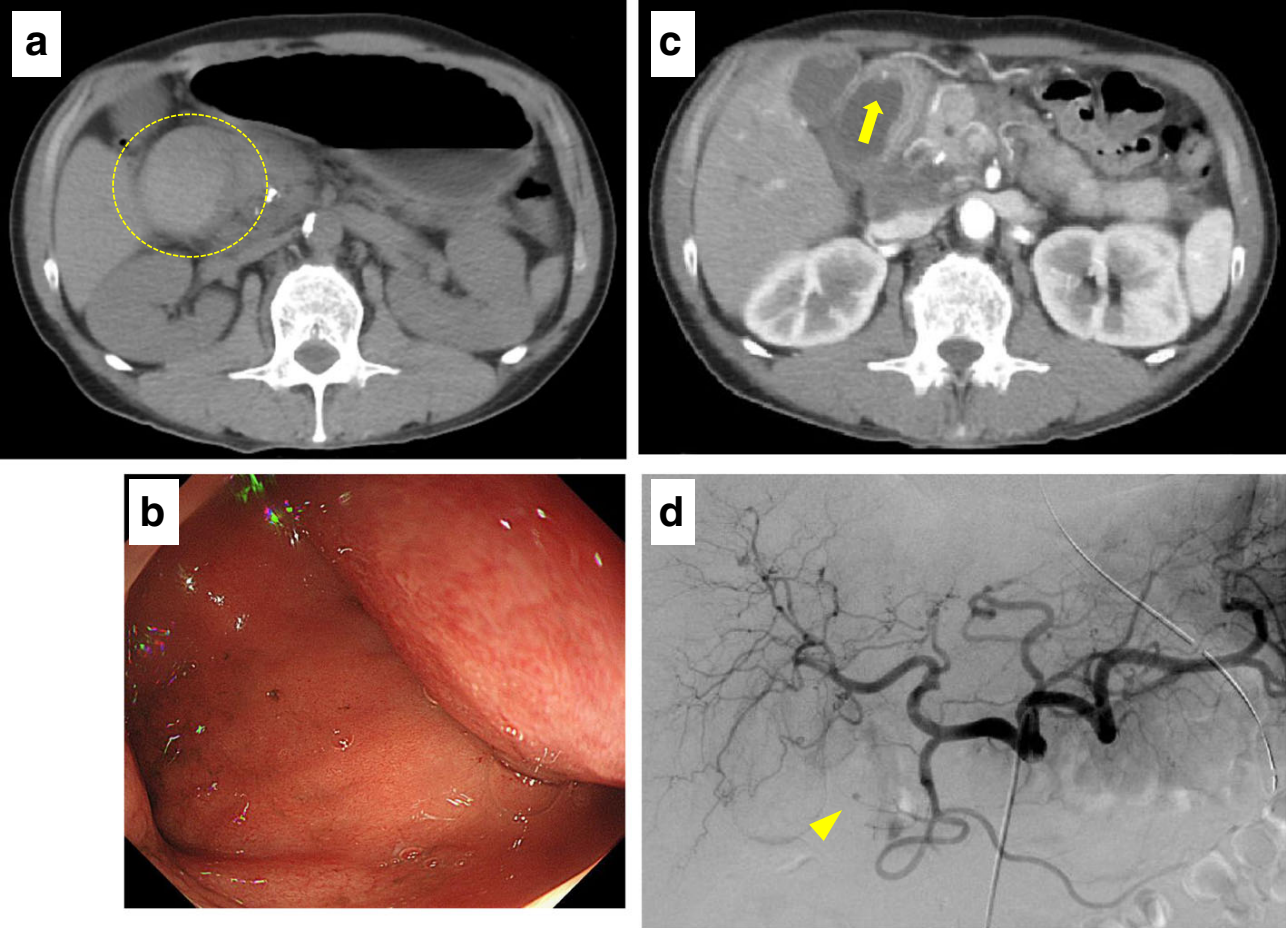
a Plain abdominal computed tomography (CT) showed a cystic lesion of 7 cm in size in the lumen near the second part of duodenum. The cystic lesion showed high density inside. b Gastrointestinal endoscopy revealed that the lumen of the duodenum was deformed by a submucosal tumor-like mass. Active bleeding was not seen in the lumen of the duodenum. c Contrast-enhanced CT demonstrated a high-density spot on the wall of the cystic lesion (yellow arrow). d A pseudoaneurysm was identified in the branch of the anterior superior pancreaticoduodenal artery (ASPDA) by angiography (yellow arrowhead)
